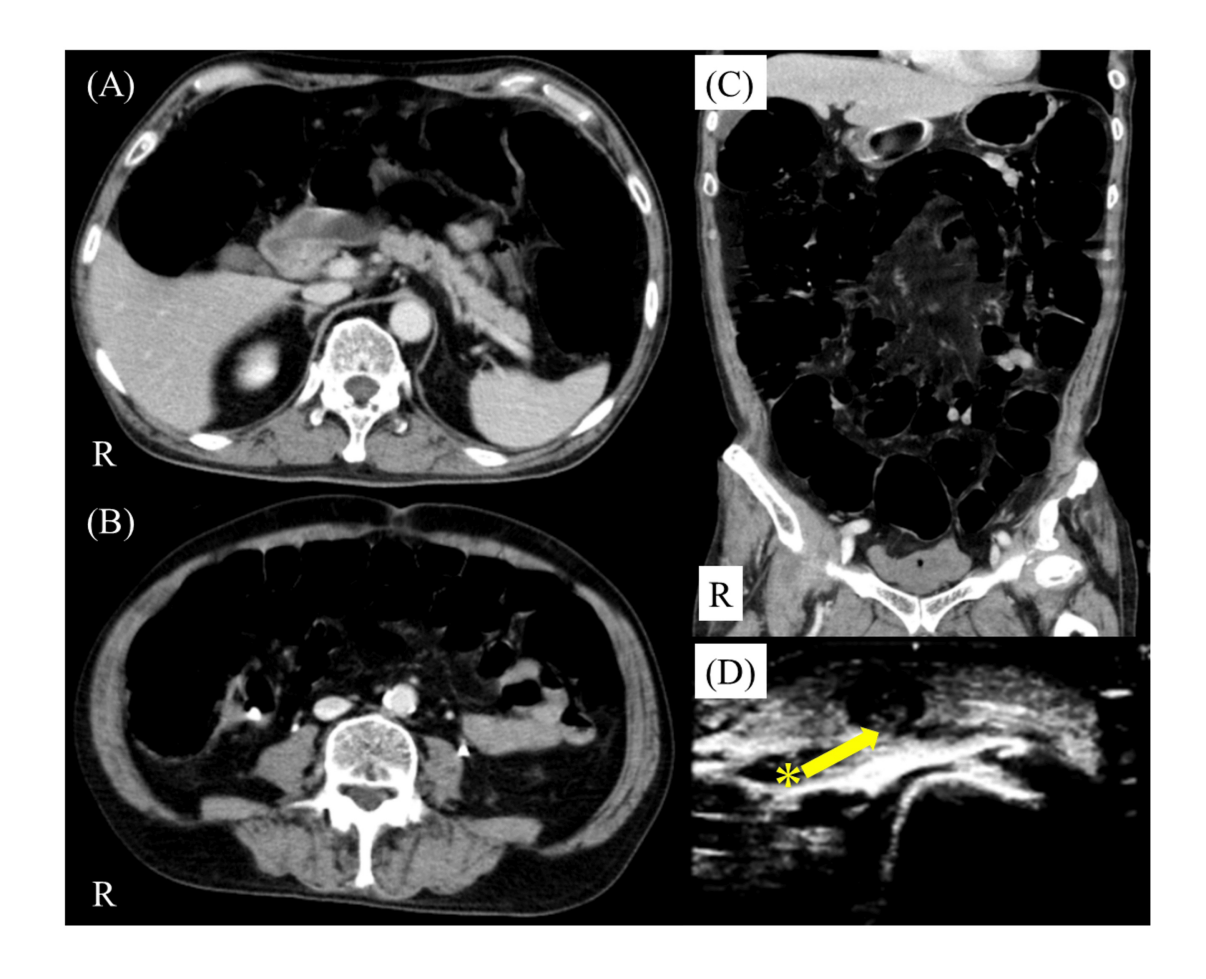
Contrast-enhanced CT scan of the abdomen (A-C) and echocardiographic image of the right forearm (D). (A) Horizontal section with cranial level. (B) Horizontal section with caudal level. (C) Coronal sections. These CT images showed gas retention and abnormal dilatation of the entire intestinal tract without obstruction. (D) A thrombus with high echogenicity was observed in the cutaneous vein (*). CT, computed tomography
